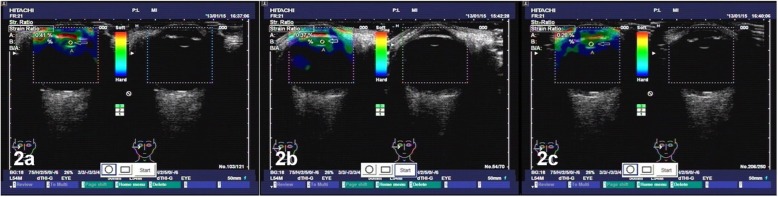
a to c Three images taken repeatedly with the ultrasound echolucency (right) and elastic (left) mode of the right eye of a 55 y/o woman from Group B with a visual acuity of 20/25 and an axial length of 23.1 mm. The dark green colour of the lens nucleus demonstrates that the strain rate is higher and therefore the lens nucleus is softer than those of the 75 y/o participant
